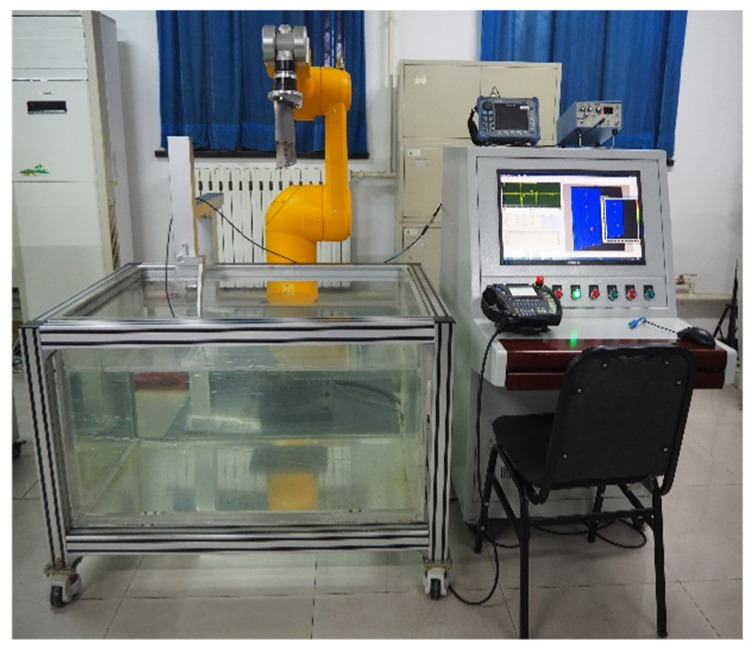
Scheme of an ultrasonic nondestructive testing system for robotics.
